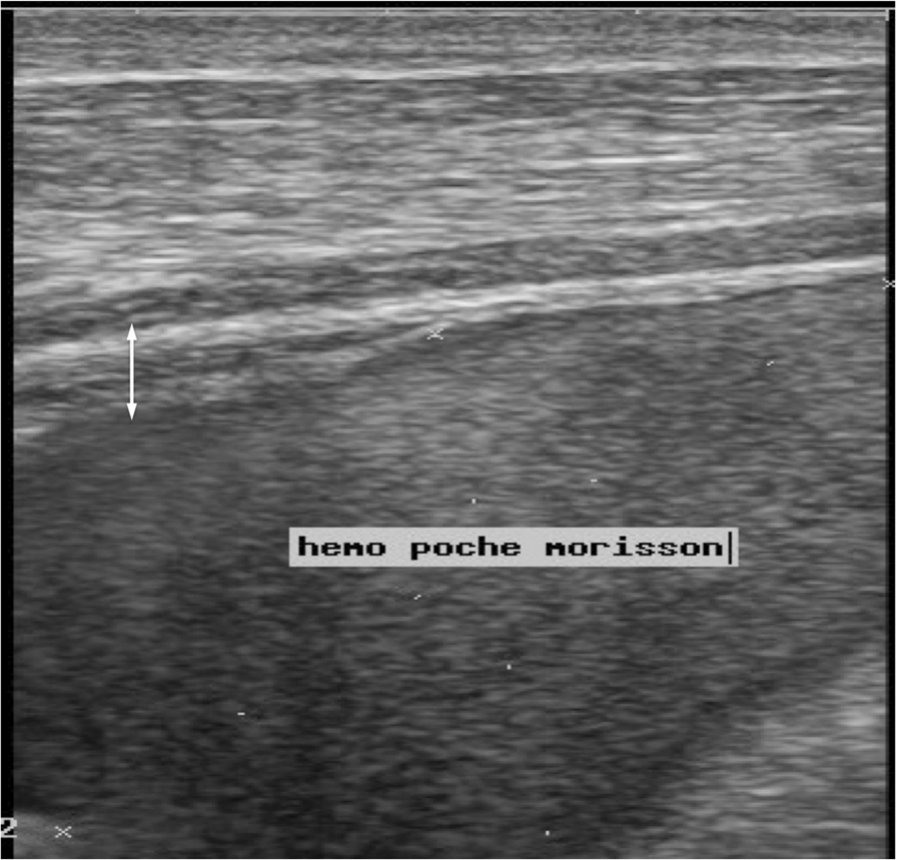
Ultrasound image of a hemoperitoneum in the pouch of Morrison with echogenic appearance of parietal peritoneum thickening (double-headed arrow)
